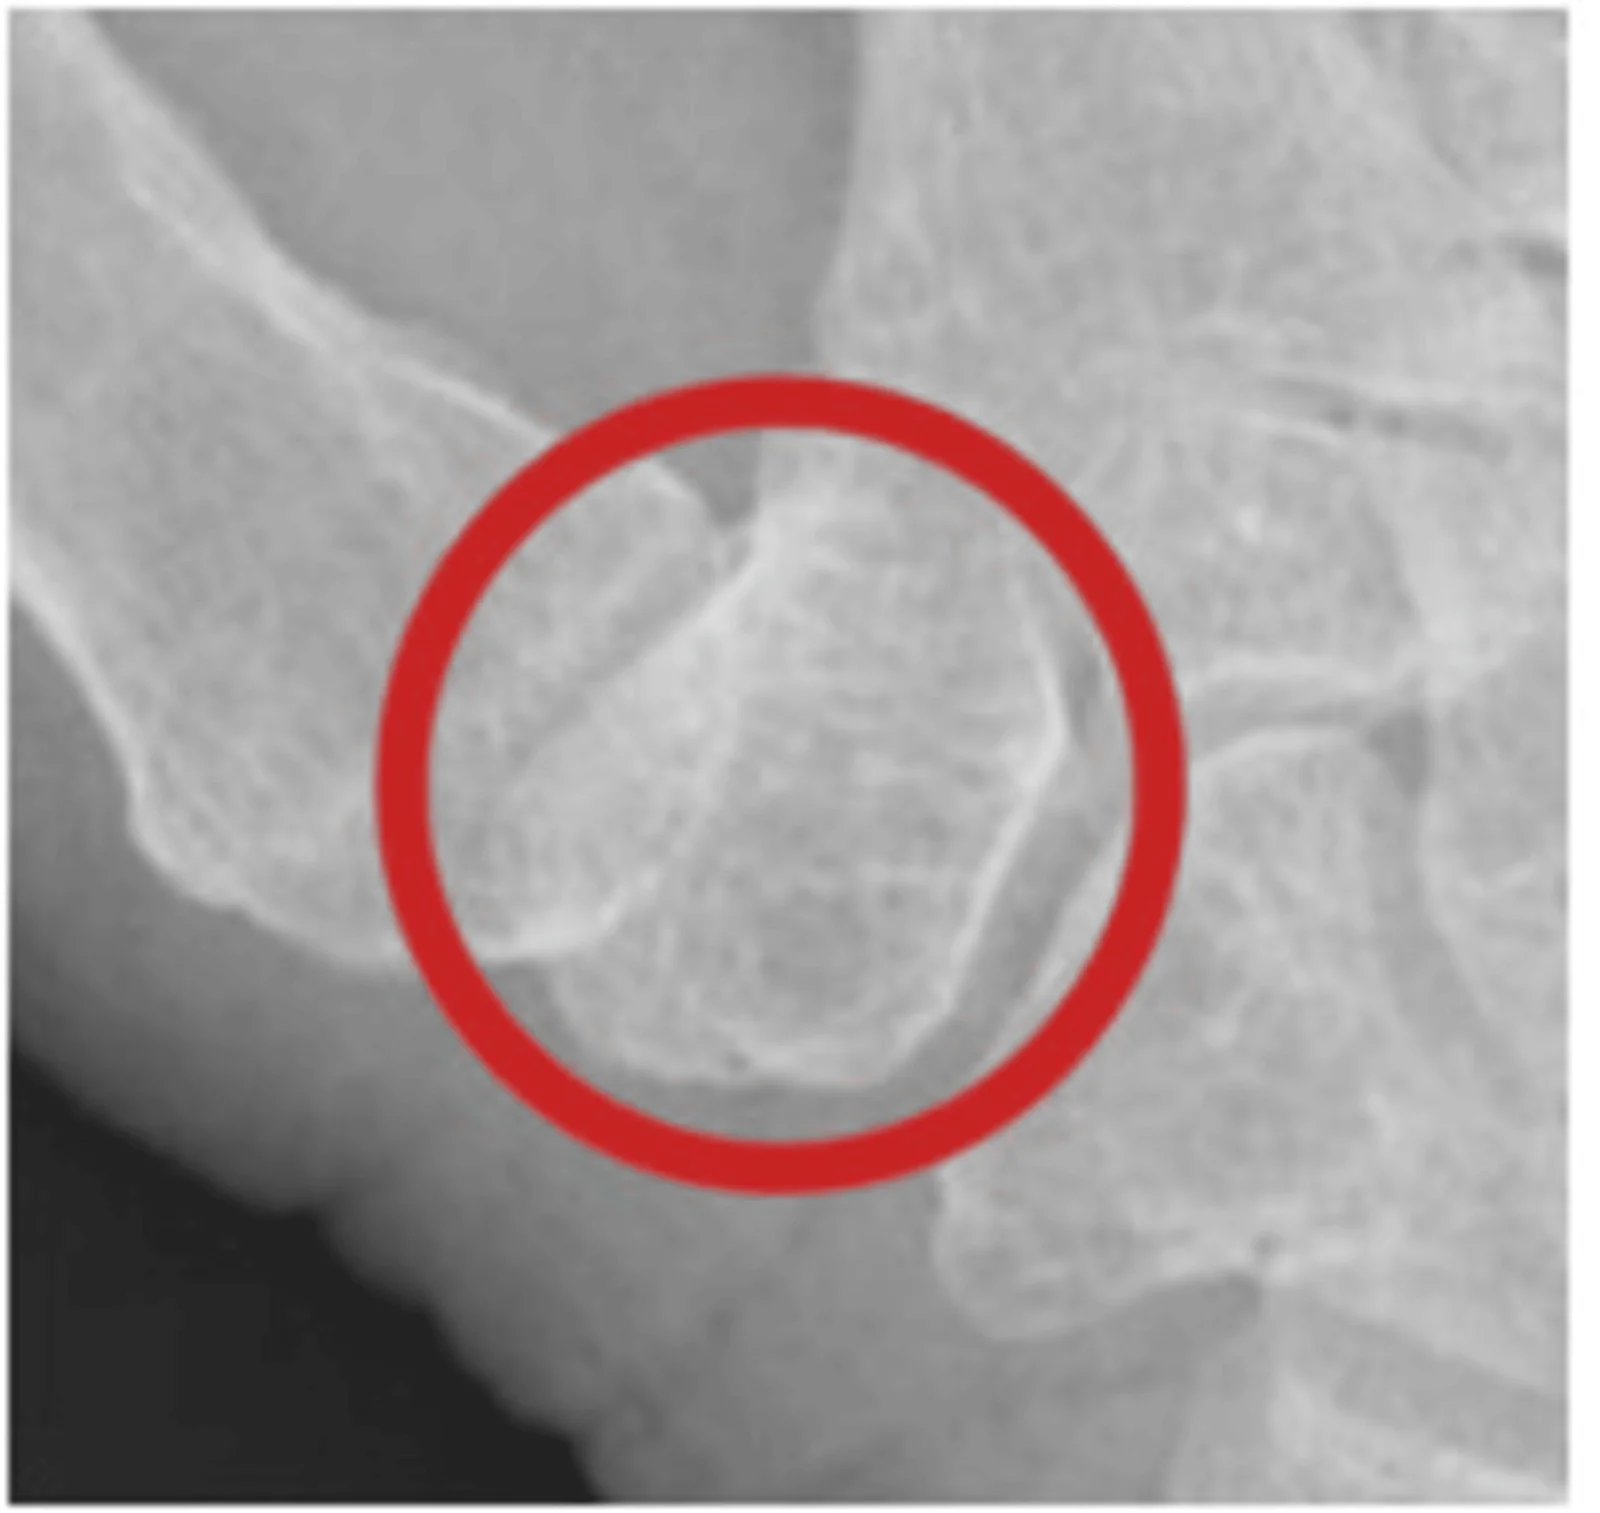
Case 2: Radiograph of the right wrist showing a fracture line suggestive of a trapezium fracture, consistent with the location of tenderness (red circle).
